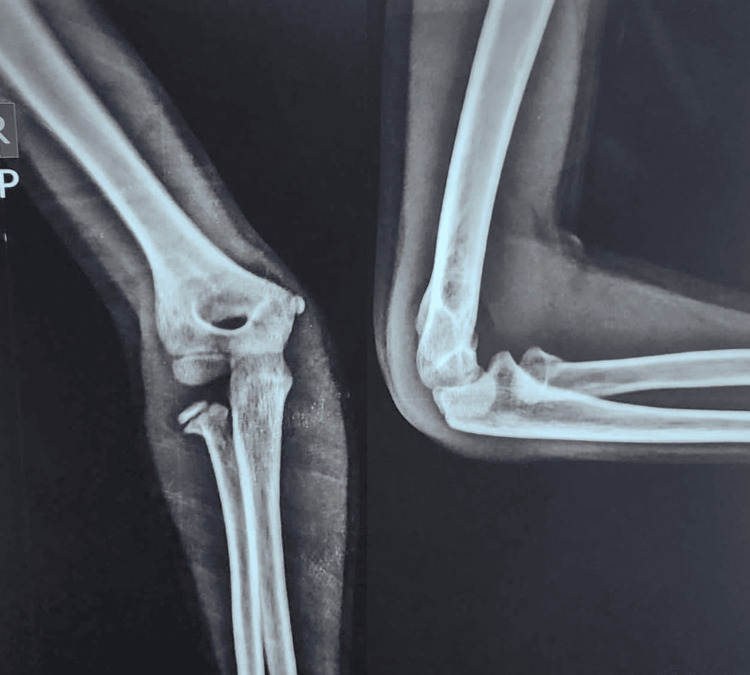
Preoperative anteroposterior and lateral radiographs showing a radial neck fracture (Judet type III)
